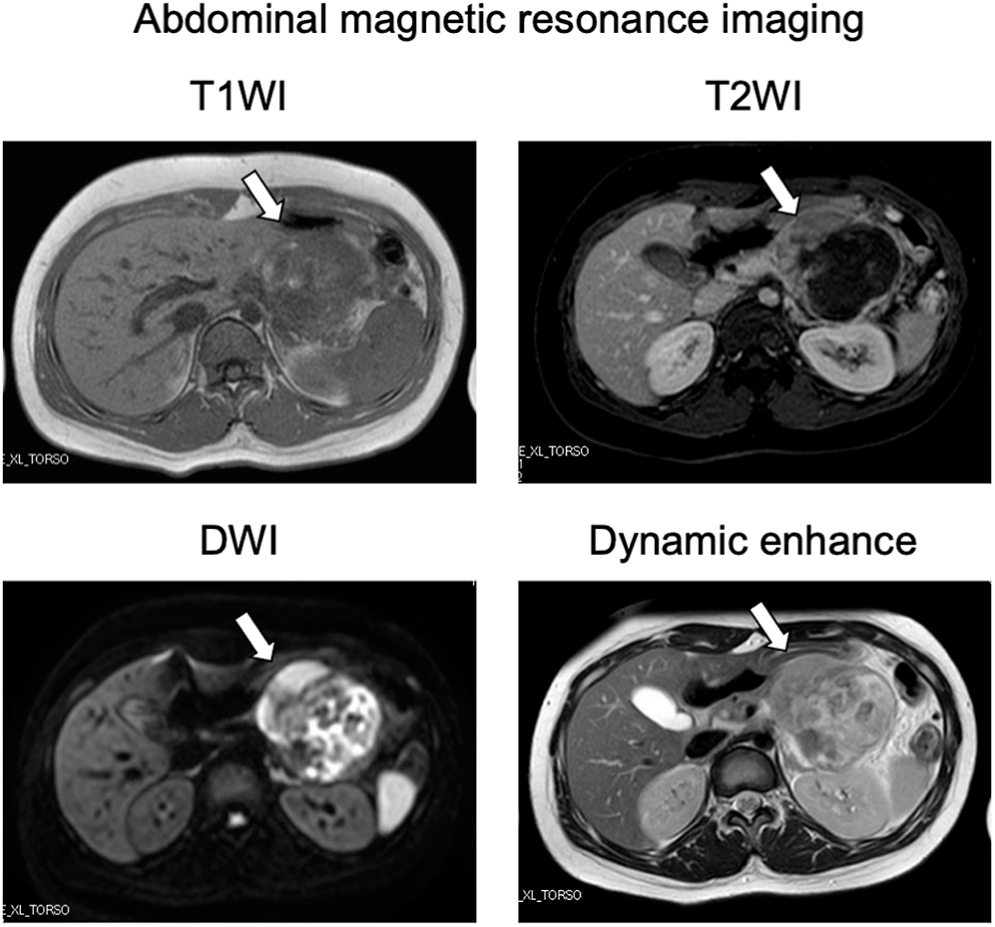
Abdominal magnetic resonance imaging (MRI): the tumor was heterogeneous, with signs suggestive of hemorrhage on T1WI. There are also findings suspicious of cystic and membrane formation, and the enhancement area with contrast effect.
